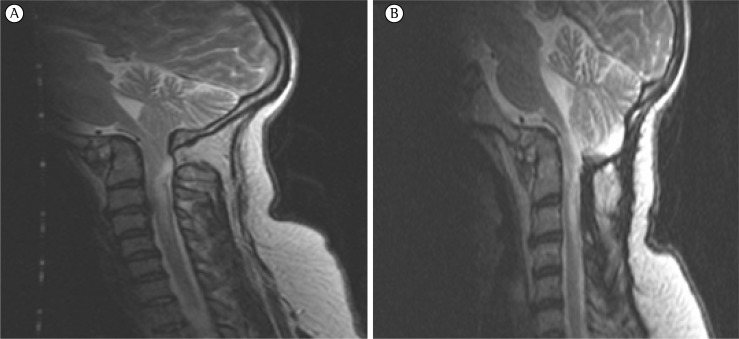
In A, presurgical magnetic resonance imaging scan of the brain showing bony
